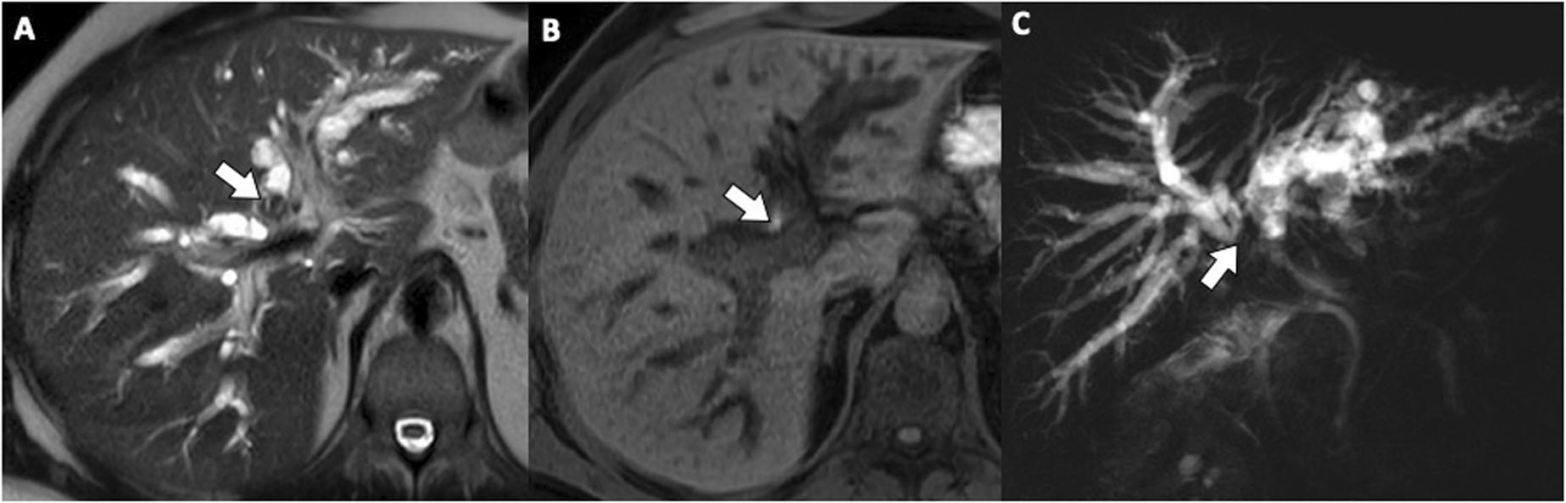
A 48-year-old man with communicating rupture. MR images on T2-weighted (a) and T1-weighted gradient echo (b) sequences demonstrate endoluminal biliary content (arrows), hypointense and hyperintense on respective images, at the hilar biliary confluence. On MRCP image (c), the endoluminal obstructive biliary content leads to loss of signal intensity (arrow) from the hilar biliary confluence to the common hepatic duct, while the common bile duct is patent
